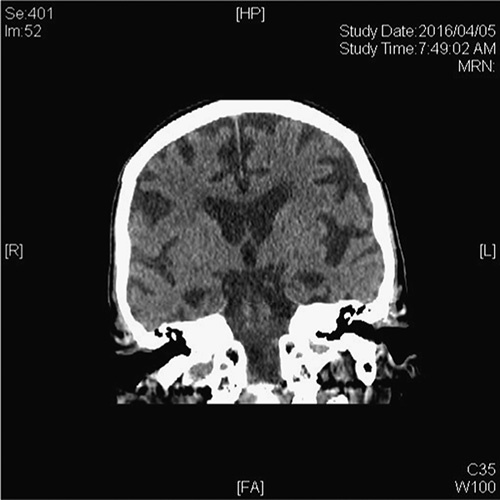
Coronal view, computed tomographic image, patient age 63, showing significant generalized atrophy and dramatic hippocampal atrophy.
